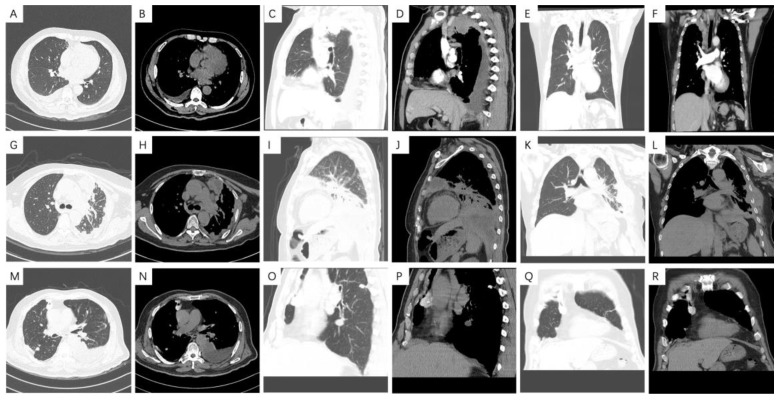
Computed tomography images in malignant pleural mesothelioma patients. (A–F) Epithelioid: A soft tissue mass shadow can be seen next to the right upper mediastinum, with a range of approximately 4.3 × 3.5 cm. The boundary between mass and mediastinum is not clear. The CT value of the plain scan was 51hu, and that of the enhanced scan was approximately 79hu. The pleura on the right side has multiple irregular thickening and obvious enhancement. There was a small amount of pleural effusion on the right side. An irregular nodular shadow can be seen in the right upper lung, with a size of approximately 1.6 × 1.5 cm. It is considered to be a malignant metastasis. (G–L) Sarcomatoid: The left pleura had uneven and obvious thickening. Soft tissue mass formation could be seen locally, and cystic changes could be seen in some lesions. Diffuse soft tissue density shadows could be seen on the left chest wall and abdominal wall, accumulating multiple ribs and thoracic vertebrae on the left side. The boundary between the lesion and pericardium, left ventricular wall, left pulmonary artery, thoracic aorta and left subclavian artery was unclear. (M–R) Biphasic: The broncho-vascular bundles of both lungs were thickened, with bronchial wall thickening, and some of them were stiff. The bilateral chest wall and pleura were extensively and unevenly thickened with multiple localized changes. Some of the tumors protruded into the lung field and some were accompanied by calcification. Multiple pleural effusions were found on both sides.
